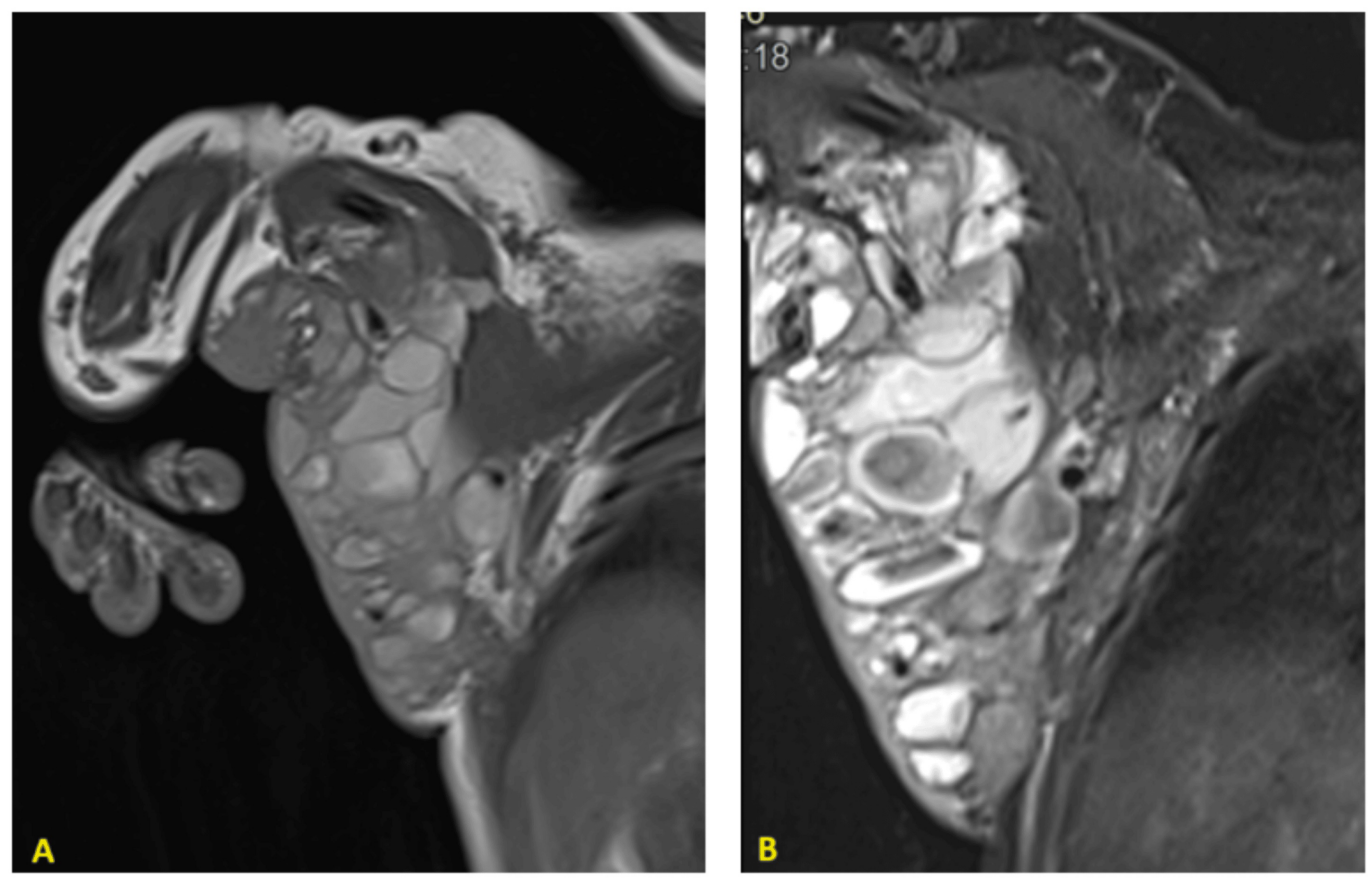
Coronal MRI Sequences of the Upper Limb and Thorax (A) T1 coronal sequence without fat saturation: multilocular lesion containing an intermediate hyperintensity, adjacent to the right lateral thoracic wall and the homolateral axillary recess (B) T2 coronal sequence with fat saturation: the loculations exhibit marked hyperintensity on T2-weighted imaging, with some showing hypointensity on T2, indicative of slow flow
